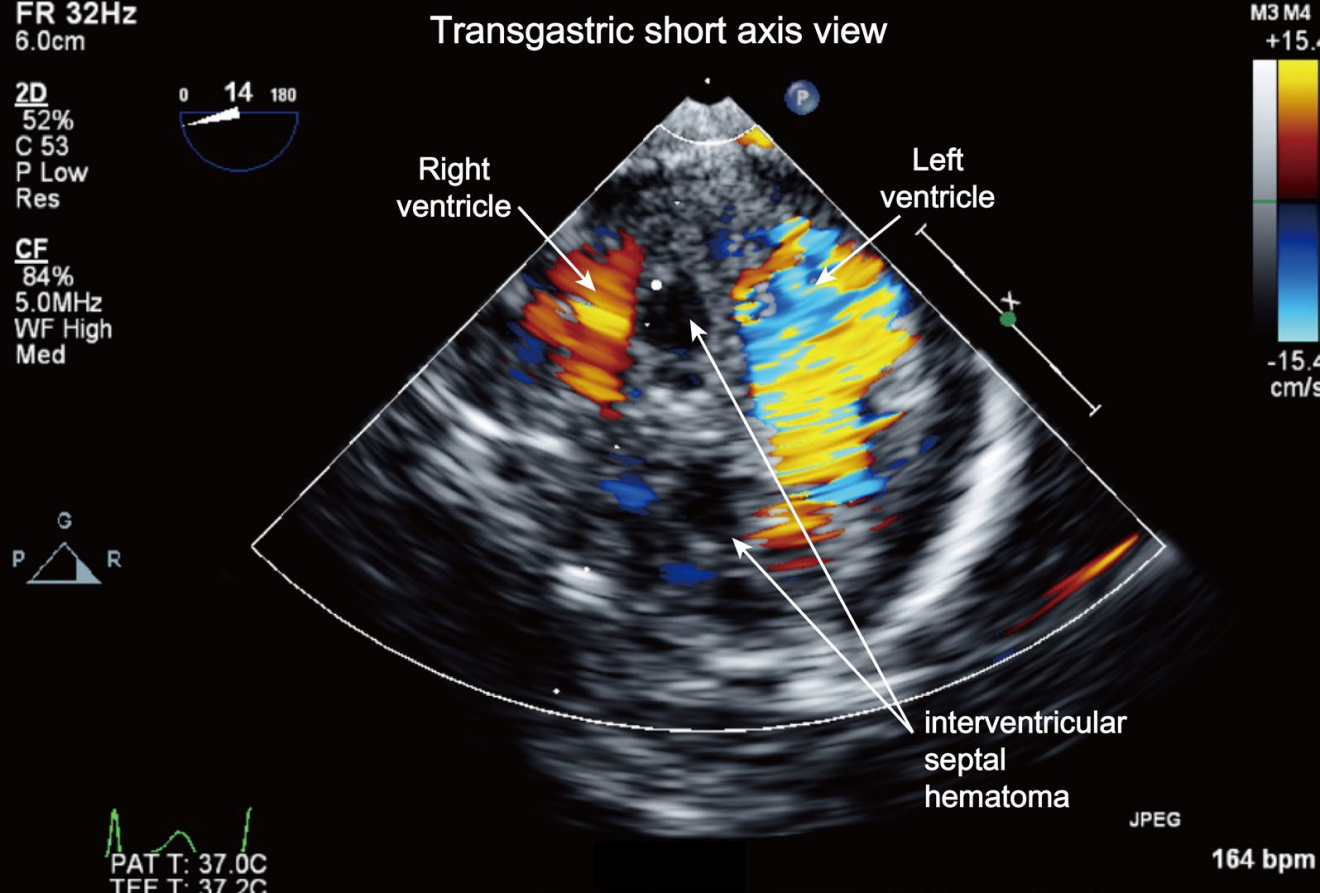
Assessing the connection between the interventricular septal hematomas and the coronary artery using color Doppler images with low Nyquist velocity limits in the transgastric short-axis view. (Additional file 2: Video SS2)
